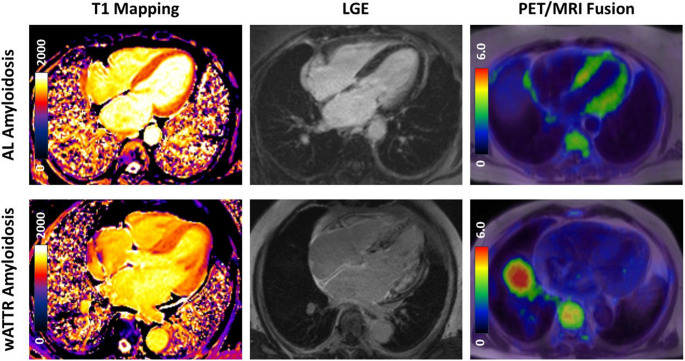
Representative case examples. (A) ^18^F-Florbetaben PET/MRI of a PET-positive, female patient with AL-amyloidosis. CMR showed abnormal mean T1-Relaxation in native T1 Mapping (1319 ms) and mild LGE, with increased ^18^F-Florbetaben uptake (SUVmax 5.6) 40–60 min post-injection. (B) negative PET of wtATTR male patient with abnormal T1 relaxation (1360 ms), strong LGE of left and right ventricular myocardium with only faint tracer uptake (SUVmax 3.3)
